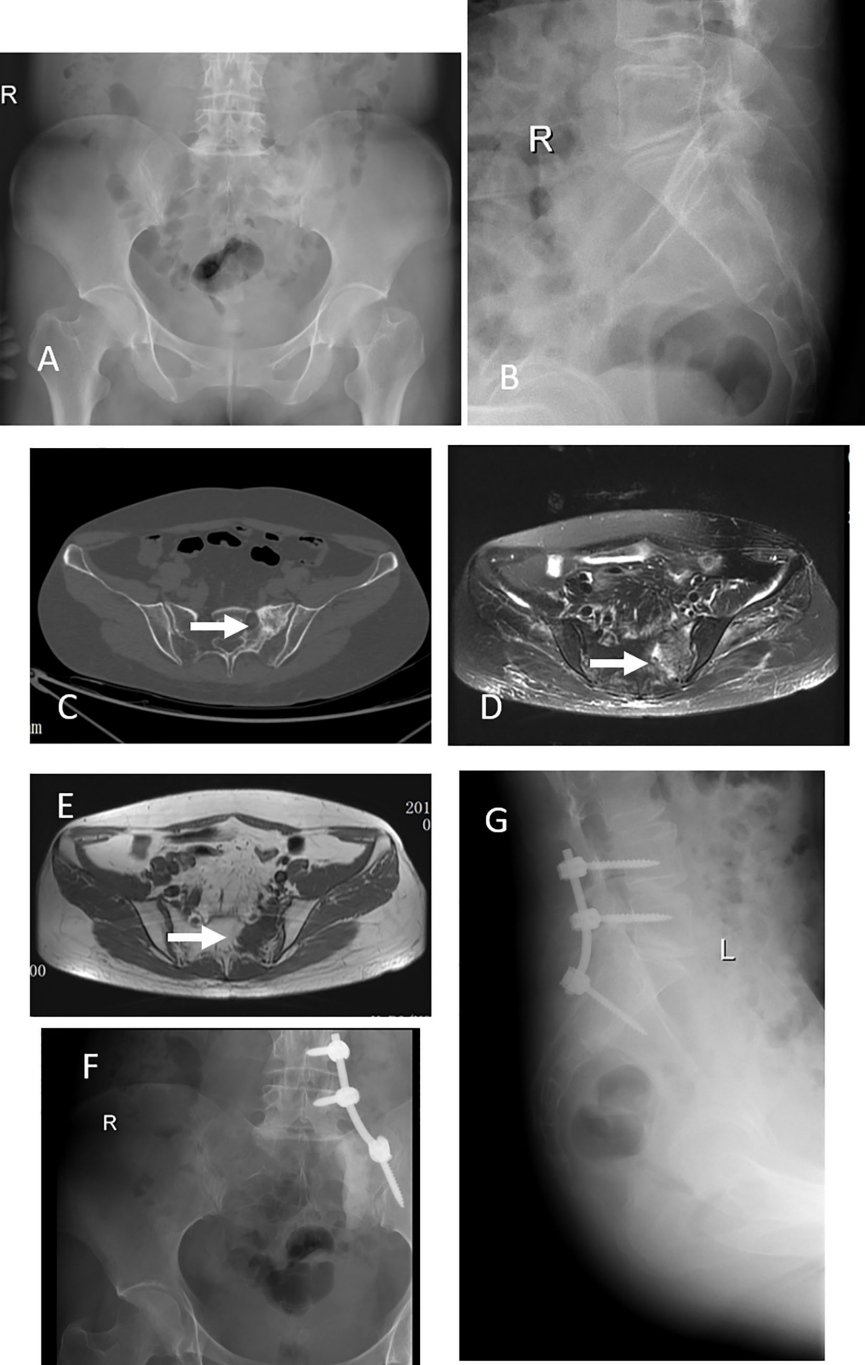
This 42 years old woman had severe back pain after breast cancer treatment for 7 years. X-ray and CT scan showed a tumor at left S1-2 (A–C), an arrow indicate the lesion. MRI scan, both T1 and T2, indicated the tumor occurred in the left S1-2 (D, E). This patient got a subtotal resection and pedicle screw fixation (F, G).
